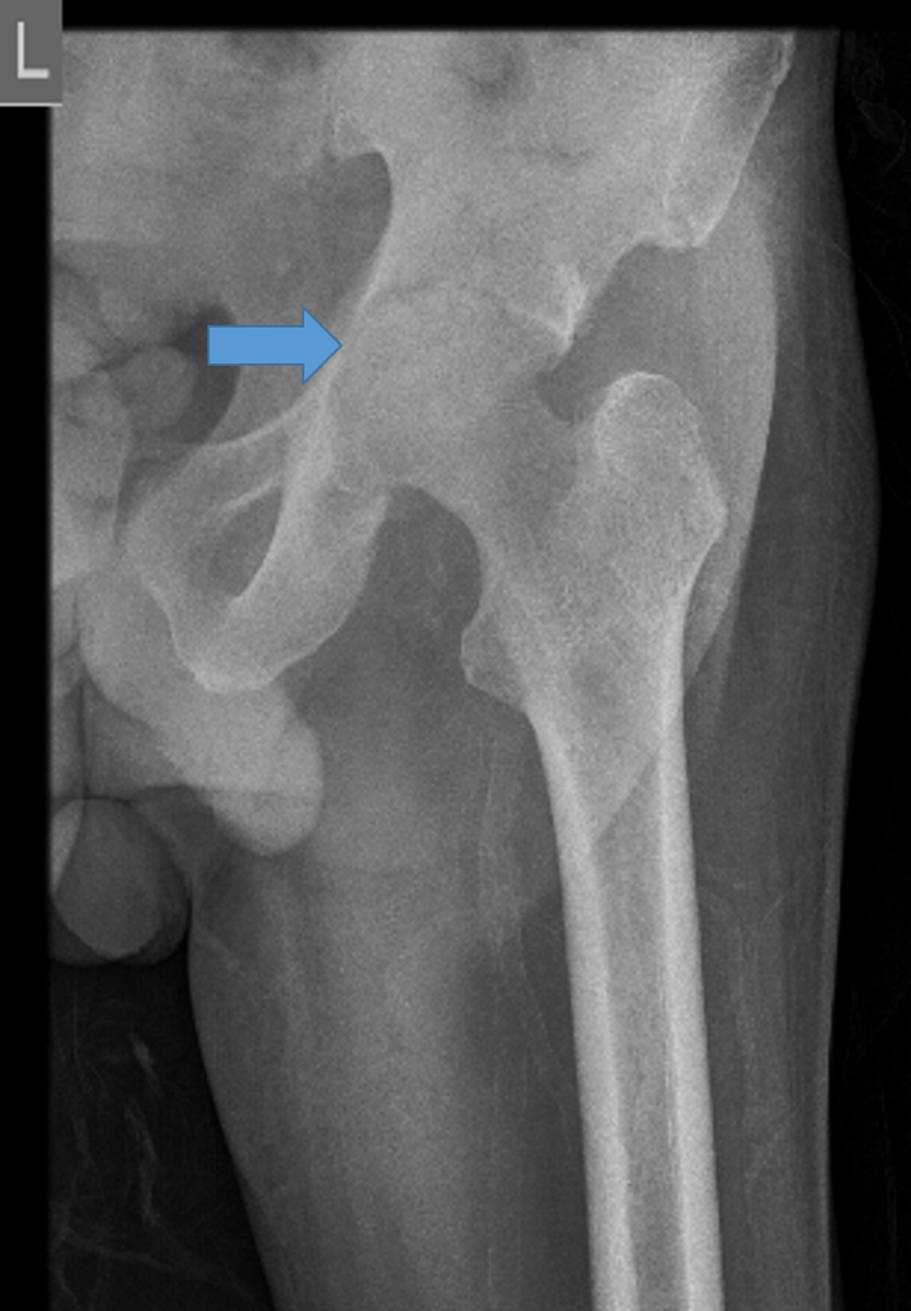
X-ray of the left hip joint showing reduced joint space.
